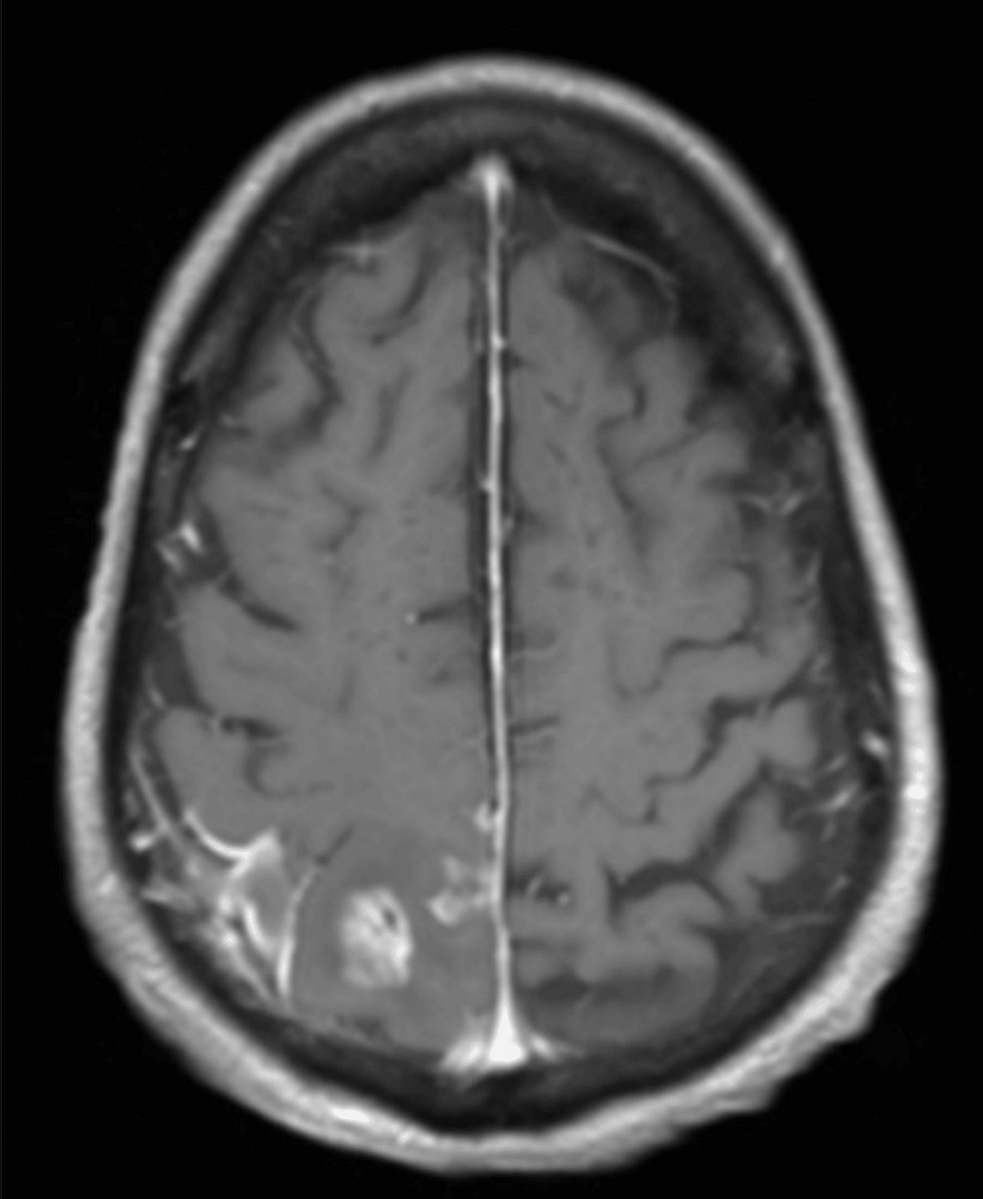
MRI with contrast, postcontrast image; worsening nodular leptomeningeal enhancement in the right parietal region with subcortical enhancing foci in the middle frontal gyrus and right postcentral gyrus MRI: magnetic resonance imaging
